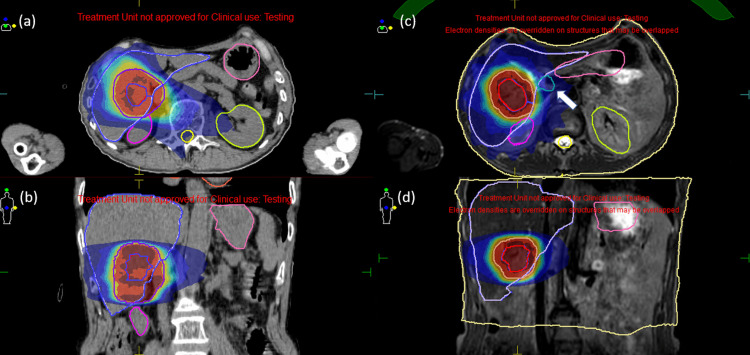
Isotoxic planning comparisons between CTgRT and MRgOART for case two. (a)-(b): CTgRT plan; (c)-(d): MRgOART plan (the first fraction). The duodenum was not adjacent to the liver in the CT image (invisible on these planes), whereas the duodenum in cobalt blue (white arrow) moved and abutted the liver at the time of MR image acquisition on the first treatment day.
